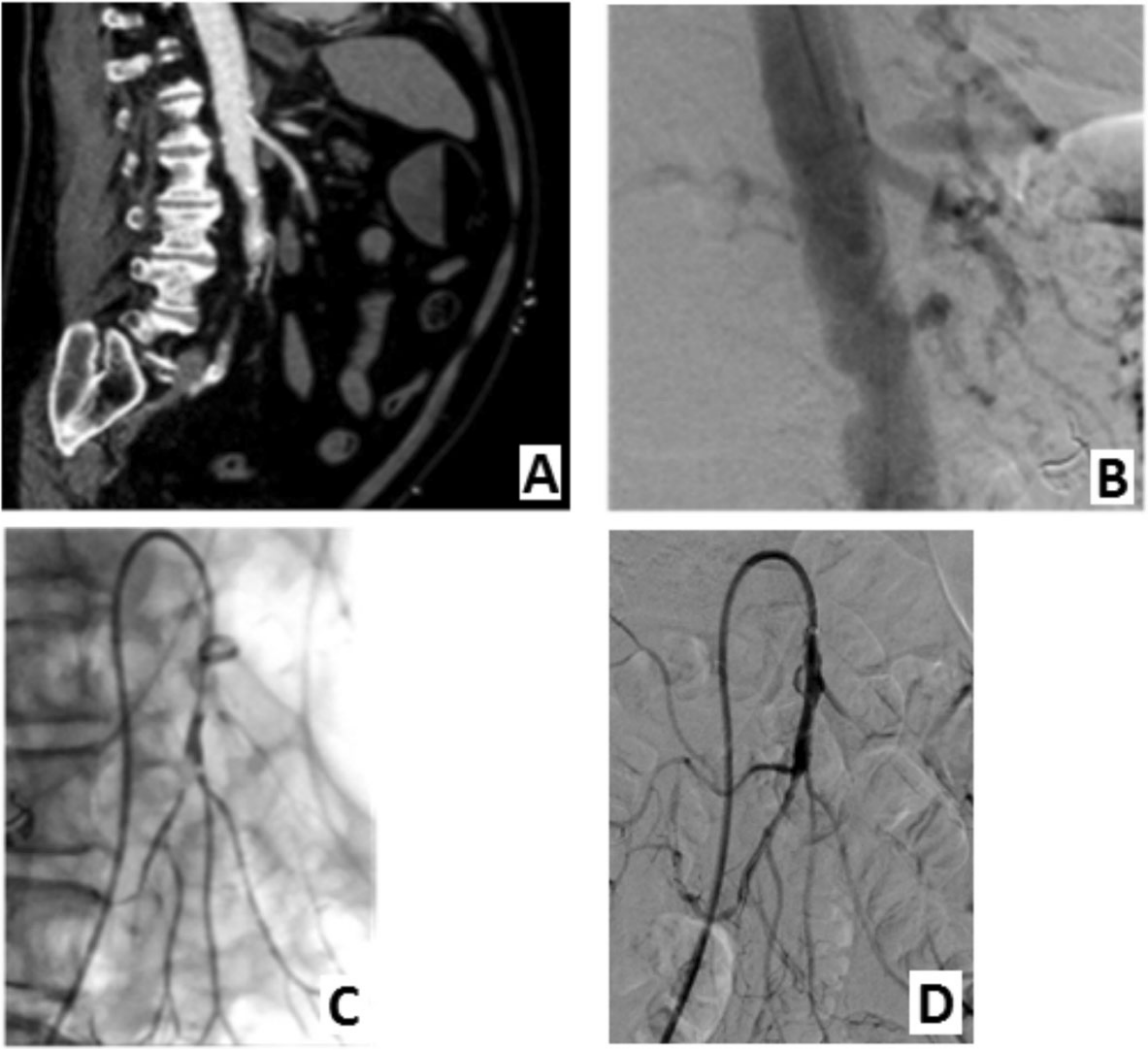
SMA embolism, occlusion main trunk and ileicolic artery (a) Sagittal CT image, arterial phase, main stem occlusion. b Lateral view DSA acute stop proximal in the SMA. c Selective SMA angiography after thrombosuction shows improved patency but residual thrombus in main stem SMA and ileocolic artery. d After PTA of ileocolic artery and main stem SMA followed by stent placement in main stem SMA shows patent SMA and side branches
